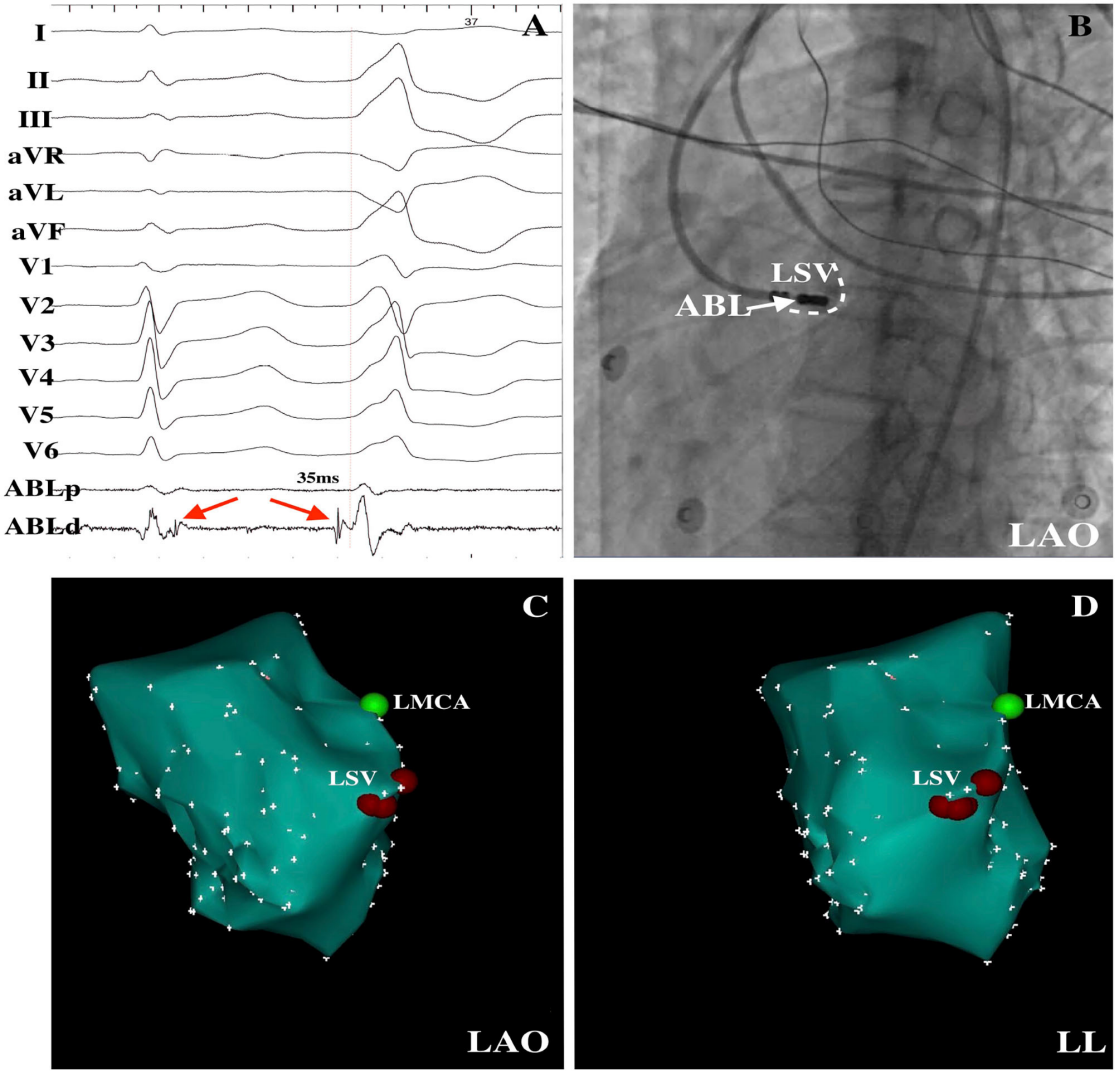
PVCs were successfully ablated within the LSV. (A) Delayed potential (red arrow) was observed during the sinus rhythm, and a DPP on the LSV preceded the QRS by 35 ms at the successful ablation site was recorded. (B) LAO radiographic view revealed the mapping catheter within the LSV. LAO (C) and LL (D) electroanatomical maps demonstrated the successful ablation points within the LSV. LSV, left sinus of Valsalva; DPP, discrete pre-potential; LAO, left anterior oblique; LL, left lateral; ABL, ablation catheter; LMCA, left main coronary artery.
